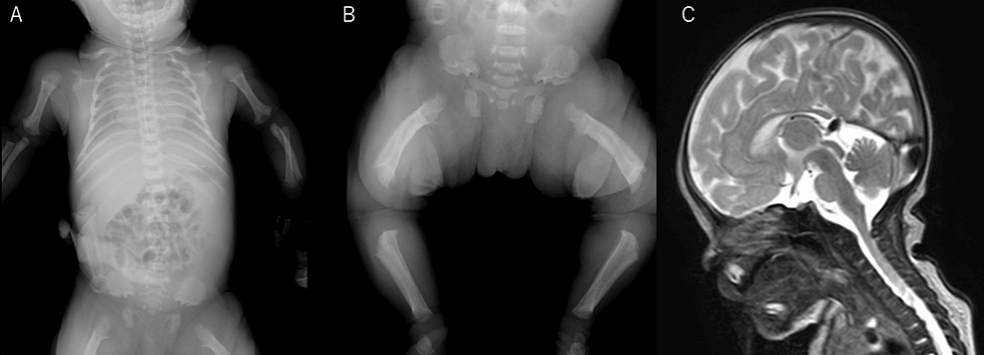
Clinical features of case 1. (A) X-ray image of the body at birth showing short, robust tubular bones, squared-off iliac wings, flat acetabula, marked narrowing of the sacrosciatic notch, and a characteristic proximal femoral radiolucency. (B) X-ray image of the legs at birth showing the same finding as in image A. (C) MRI at eight days of age showing mild foramen occipitalis magnum stenosis.
